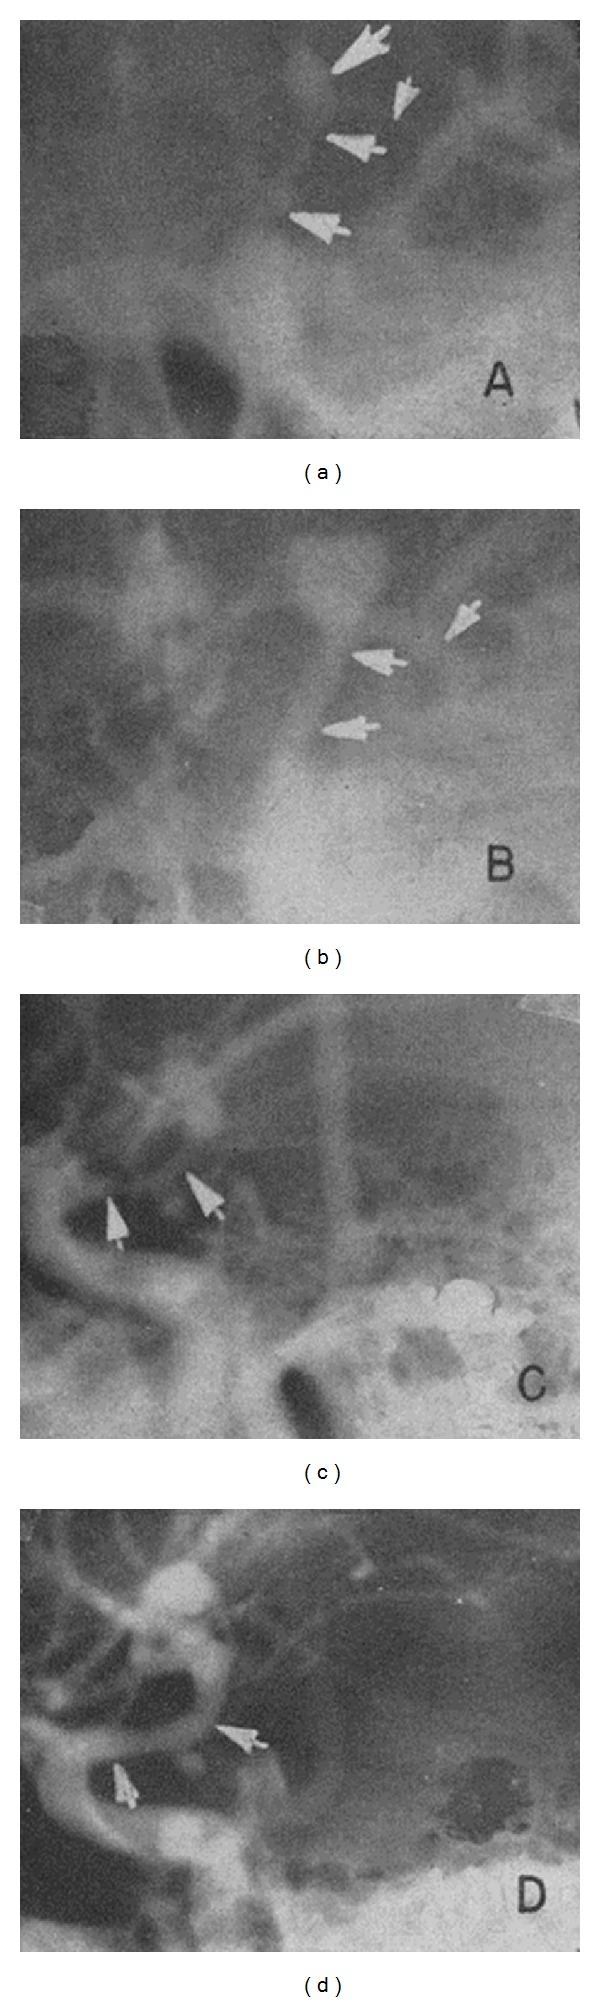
First radiographic demonstration of cerebral vasospasm at bifurcation of left ICA using arteriography adapted from [2].
